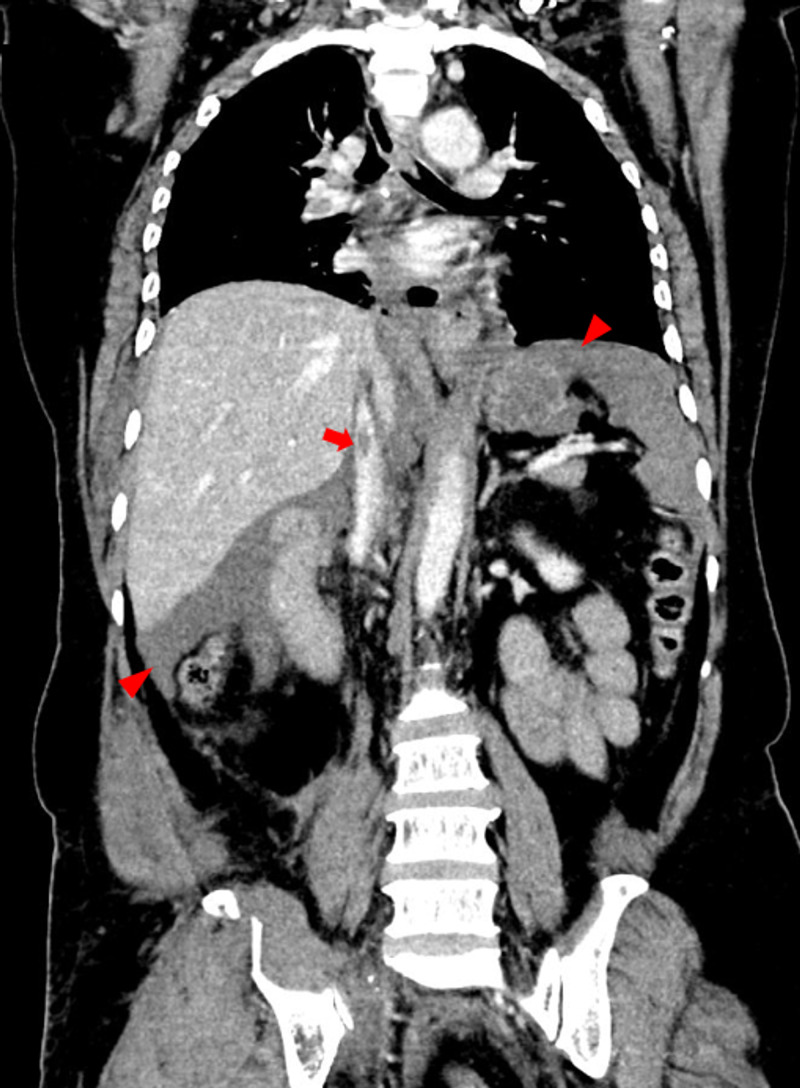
Coronal image of the contrast-enhanced computed tomography (CECT) of thorax, abdomen and pelvis showing a partial thrombus in the infra-hepatic inferior vena cava (arrow). Mild fluid collection seen in the perihepatic and perisplenic spaces (arrowheads) indicating presence of hemoperitoneum.
